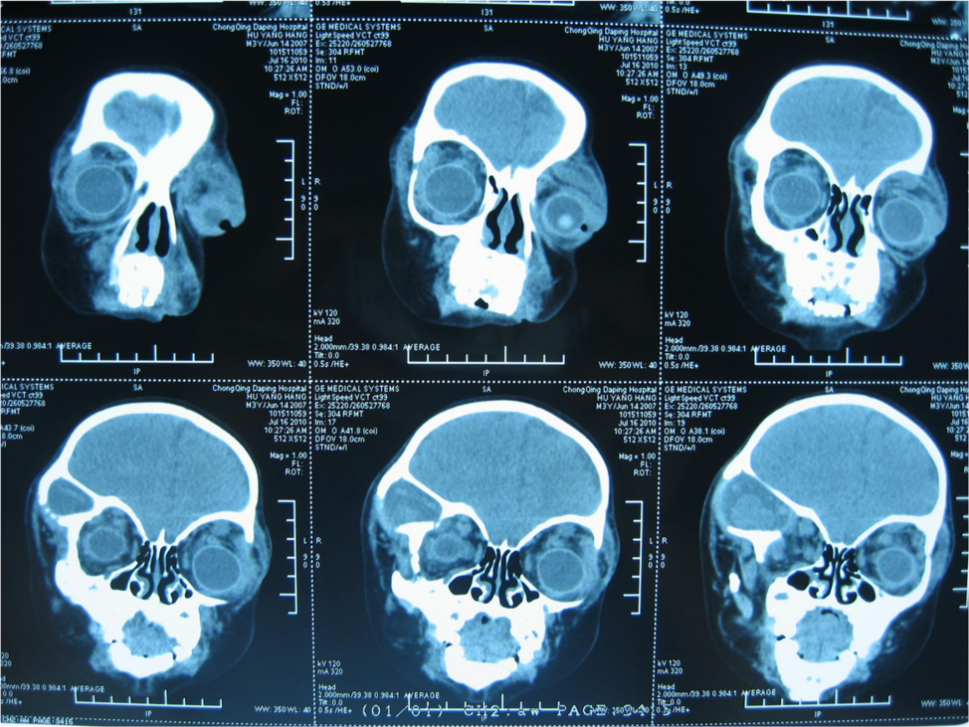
A coronal T2-weighted MRI shows focal areas of high signal intensity in the head and orbit. These may be the result of gliosis or abnormal myelination. And the sphenoid wing dysplasia is also present
